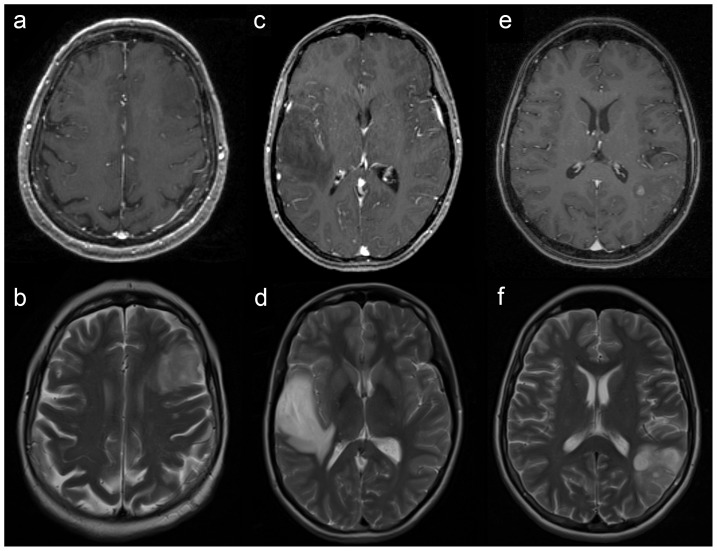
Pattern of contrast-enhancement (CE) on magnetic resonance imaging (MRI) of gliomas with non-significant CE. T1-weighted contrast-enhanced MR images demonstrate examples of gliomas with (a) no visible ( = *none*) CE, (c) unspecific ( = *patchy/faint*) CE and (e) a small regional ( = *focal*) CE in an otherwise non-enhancing tumor. (b,d,f) T2-weighted MR images show the corresponding hyperintense glioma lesions.
